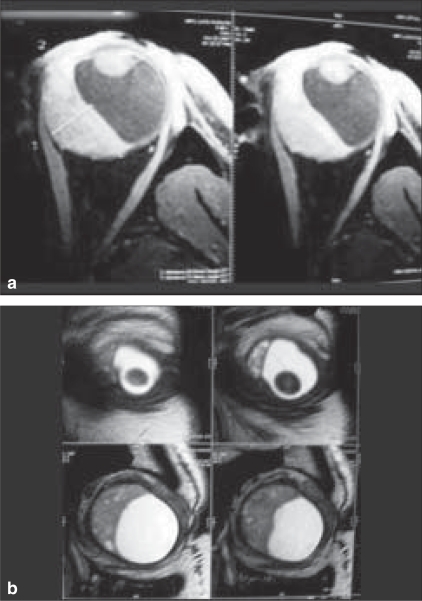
The magnetic resonance imaging findings
